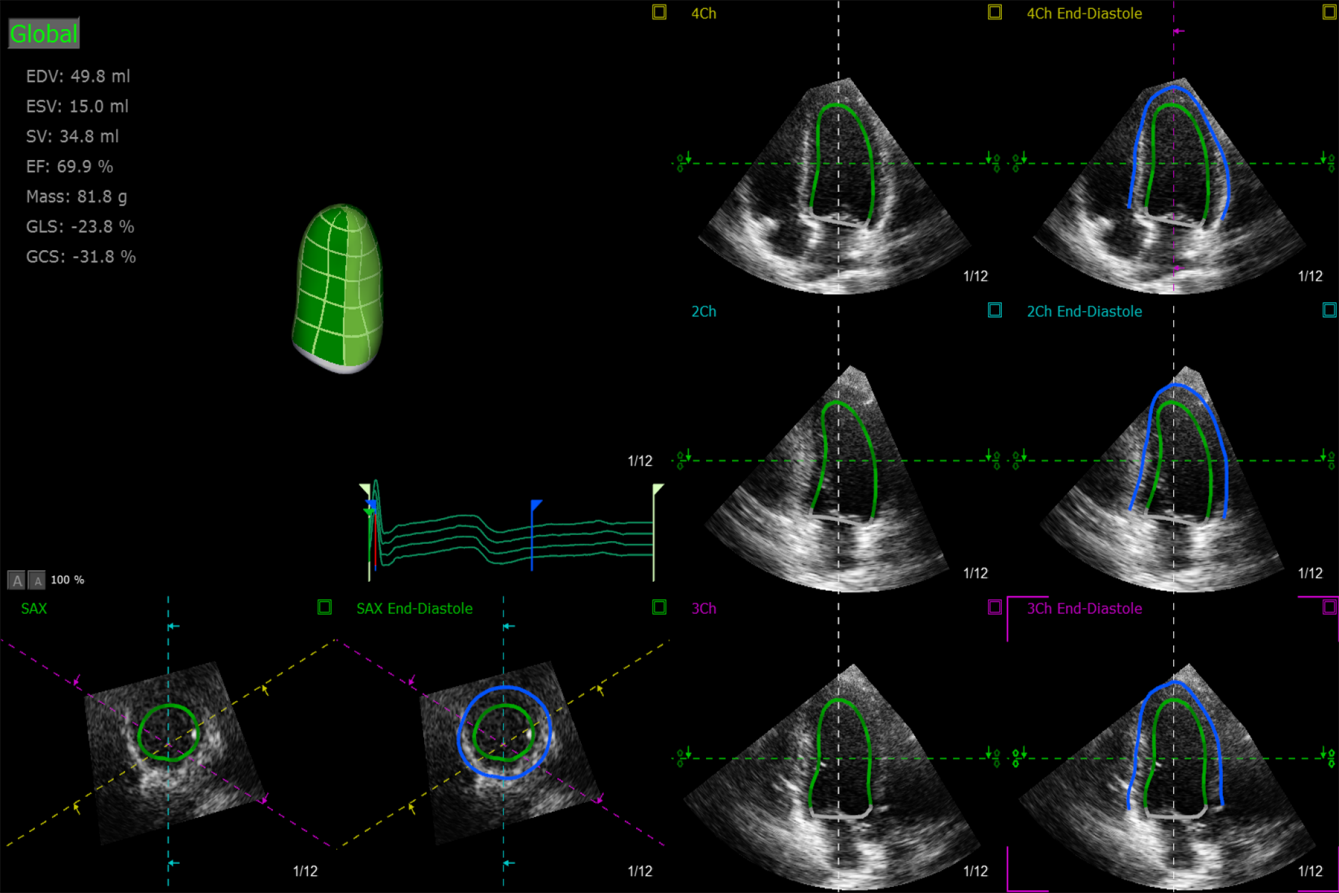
Offline analysis using 3-dimensional speckle tracking echocardiography. (A) Semi-automated endocardial border (*solid green line*) identification and tracking. (B) Semi-automated epicardium border (*solid blue line*) identification and tracking.
